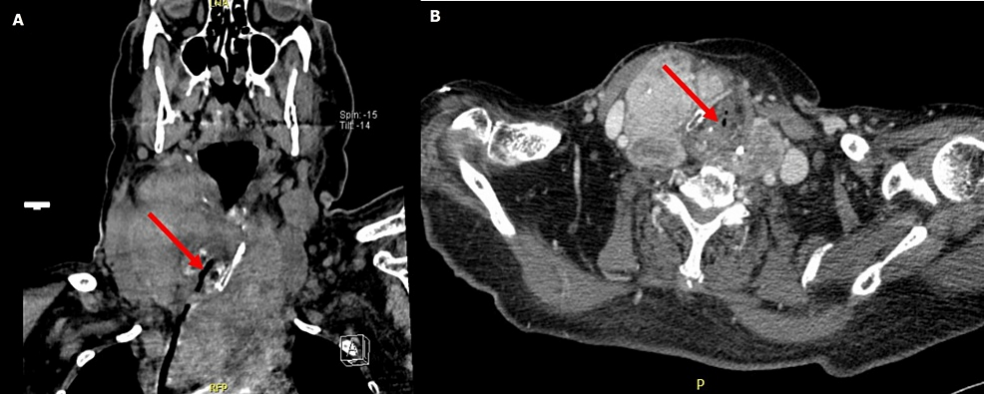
Neck and thoracic CT scan (coronal and axial) showing massive vascular and airway compression by substernal goitre. Trachea location is identified using red arrows.
